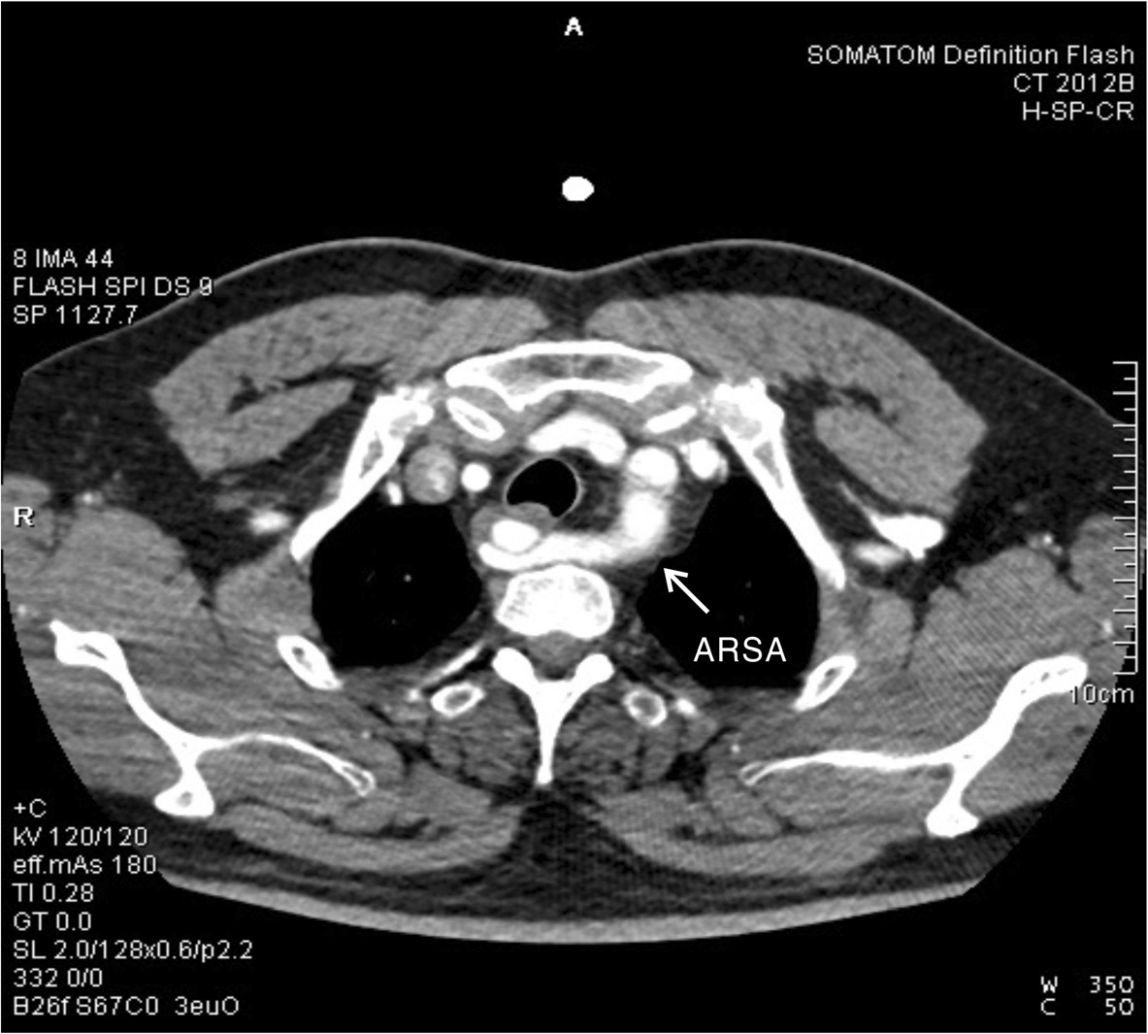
Computed tomography shows a Stanford type B aortic dissection involving the aberrant right subclavian artery
